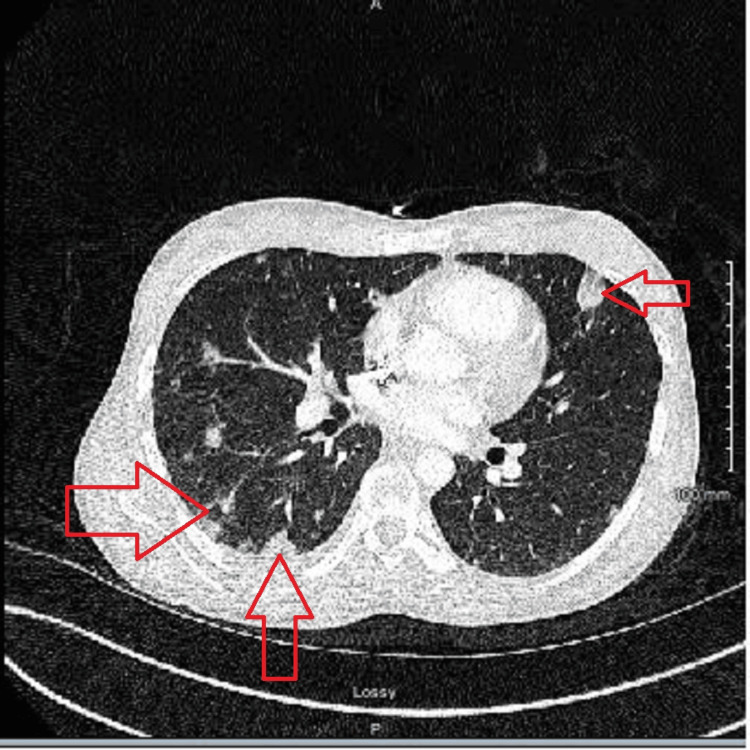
CT of the chest showing extensive septic emboli in the lungs.
